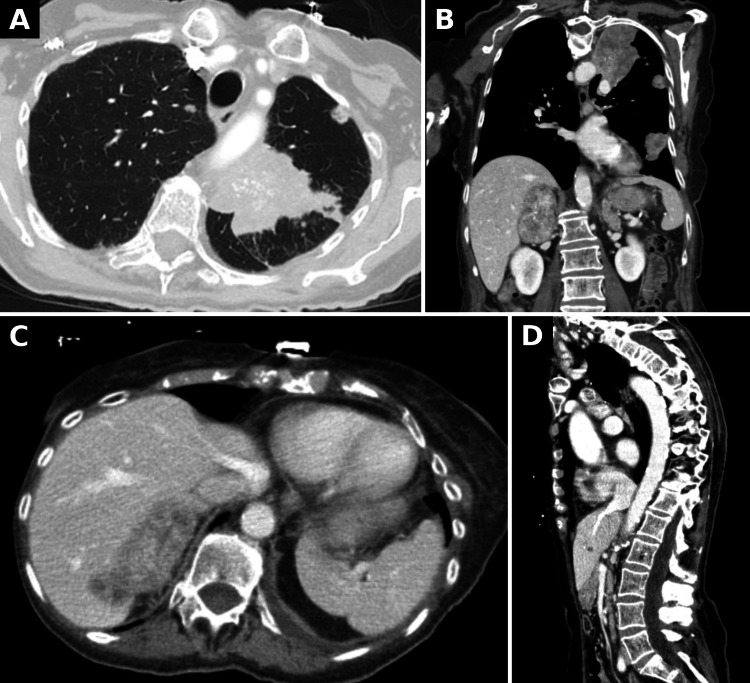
Computed tomography (CT) of the chest, abdomen, and pelvis demonstrating a left upper lobe pulmonary mass (A), bilateral heterogeneous adrenal masses (B), hepatic lesion (C), and thoracic spine lytic lesions (D)
